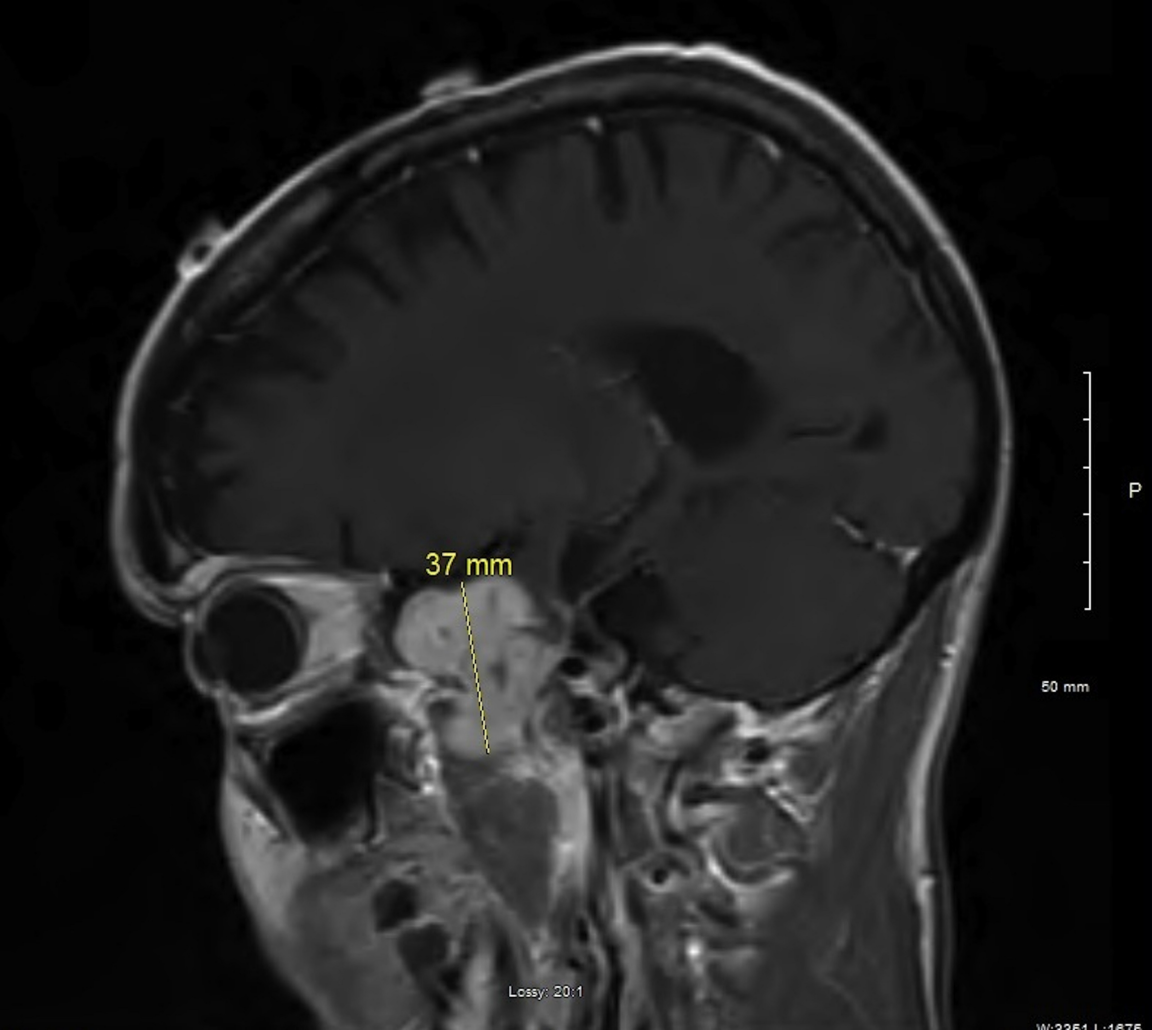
Brain MRI, Sagittal View Without Contrast Enhancement A large temporal lobe mass bridging the skull base into the left pterygomaxillary region.
